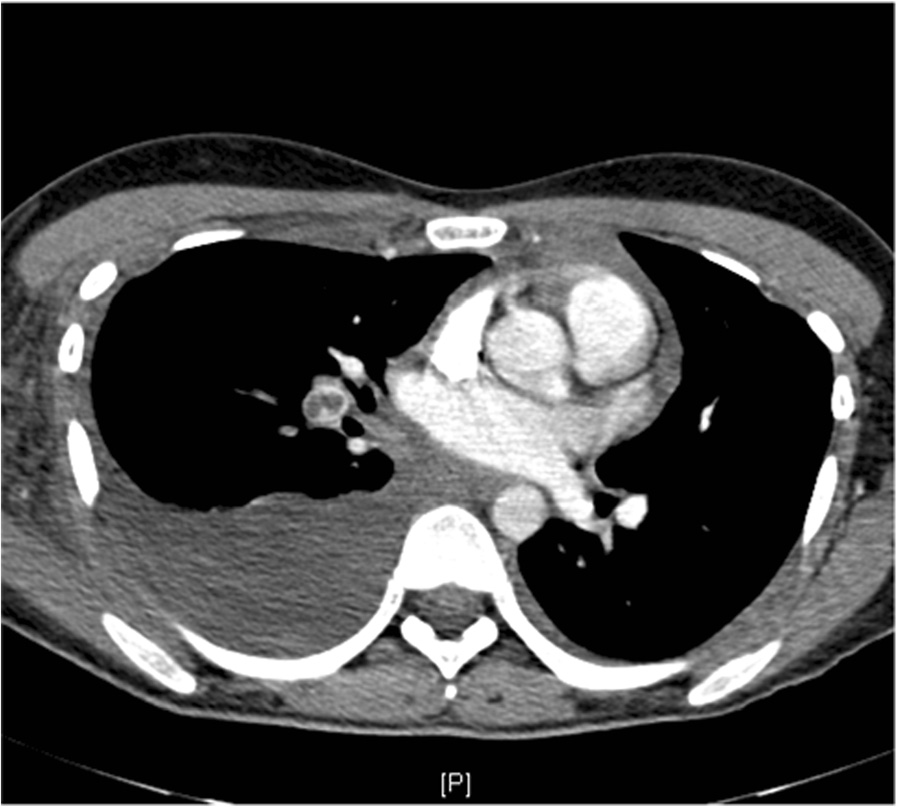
Computed tomography scan of the thorax demonstrating an acute thrombus within the right lower lobe pulmonary artery in keeping with a pulmonary embolus and a large right pleural effusion.
